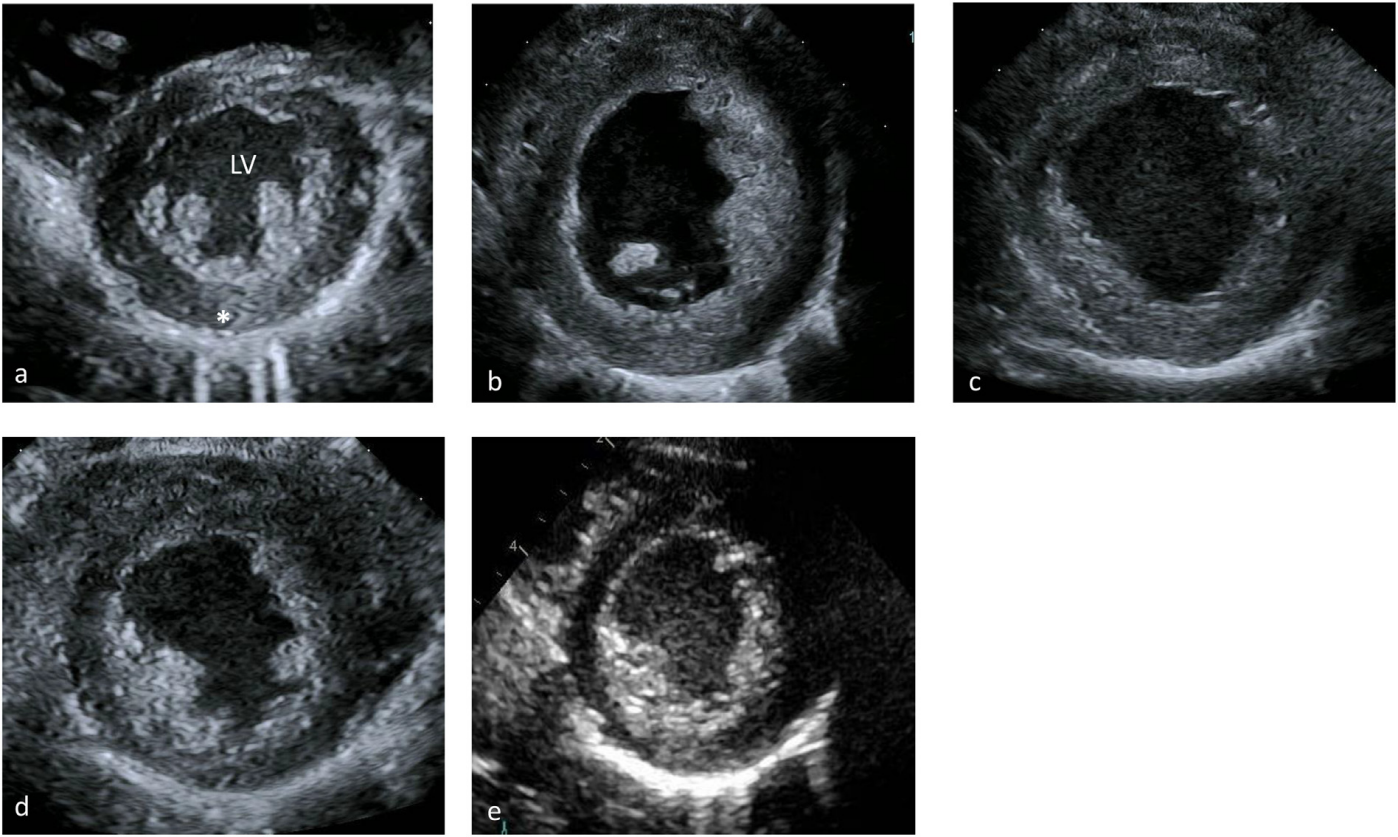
Parasternal short axis echocardiographic view of a (a) normal heart with a similar view of this patient's hearts at (b) time of diagnosis (c) two months after initiation of ERT (d) four months after initiation and (e) a year after initiation. These images emphasize the thickness of the left ventricular wall and improvement during the course of treatment. LV left ventricle, asterisk is on the left lateral free wall of the left ventricle.
